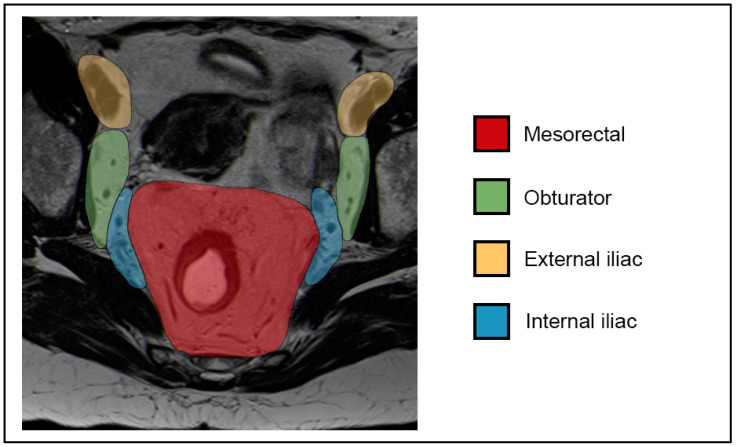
T2-weighted axial MR image: mesorectal, obturator, external, and internal iliac lymph node compartments.
